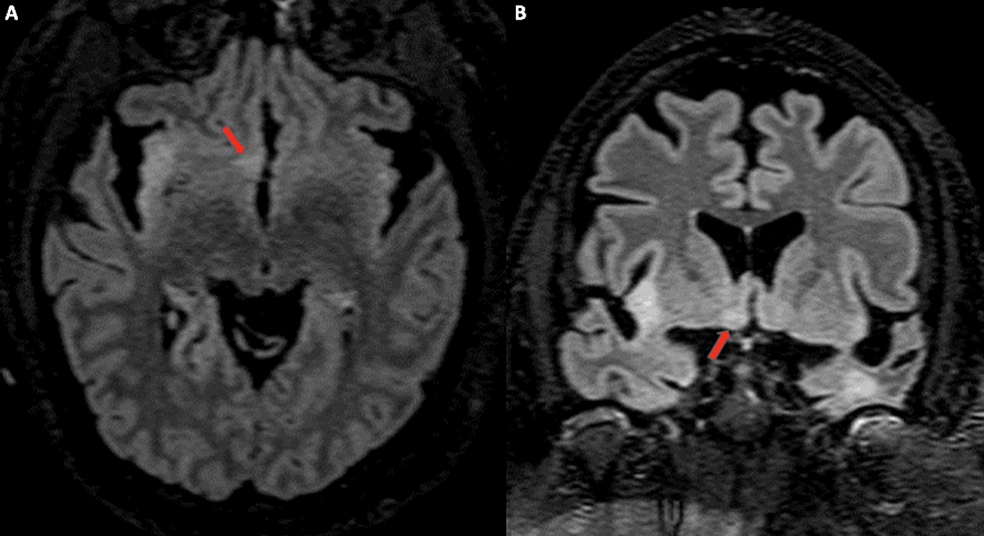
Axial (A) and coronal (B) T2 FLAIR MRI obtained in 2023 Demonstrates new hyperintensity in the right hypothalamus (arrow), persistent hyperintensity in the right insula and left temporal lobe, and severe mostly perisylvian atrophy.
